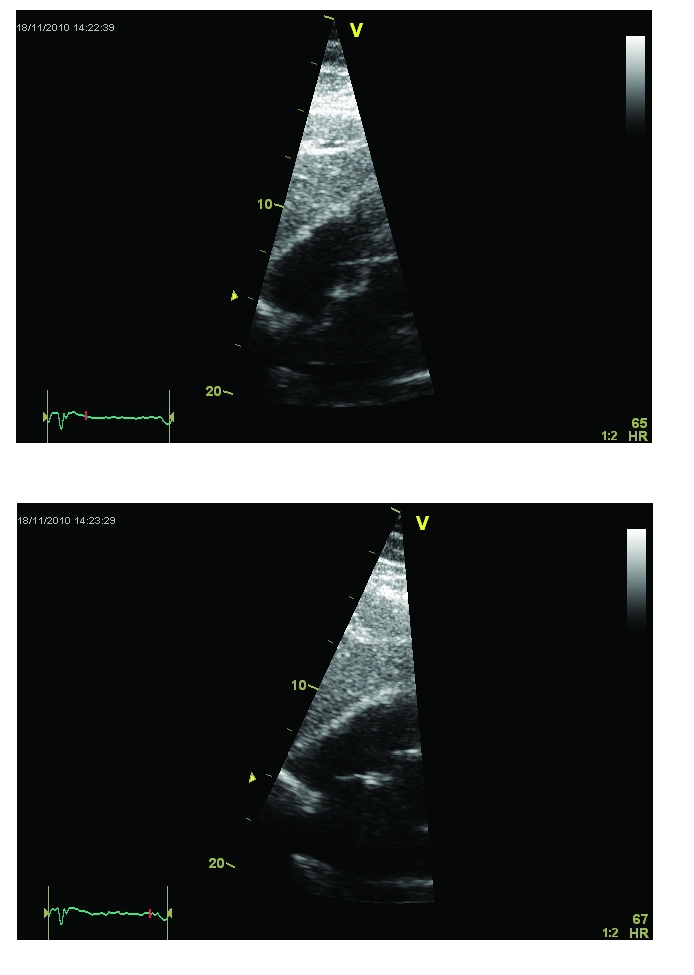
Bidimensional transthoracic echocardiography from the subcostal view. Systolic (A) and diastolic (B) frames showing the ample excursion of the interatrial septum, suggesting the presence of a septal aneurysm.
